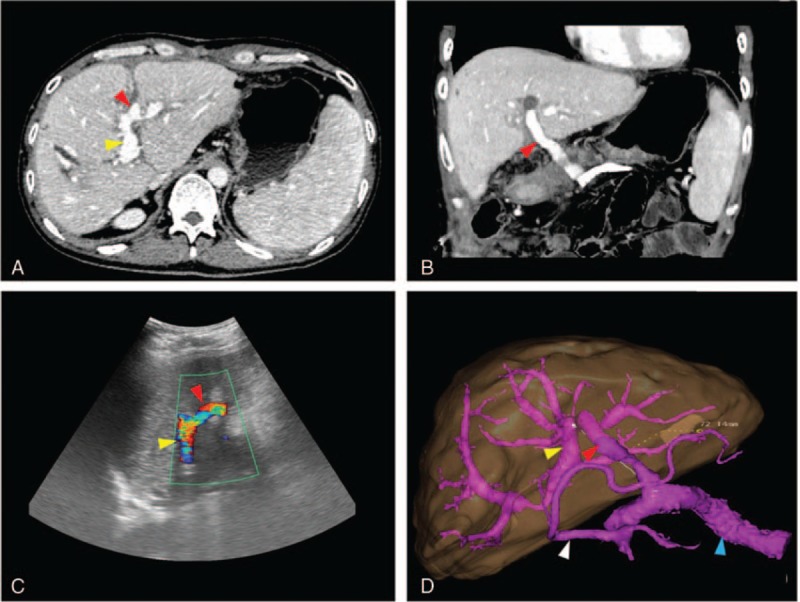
Postoperative enhanced CT scan (A, B) and Doppler US images (C) showed a patent LPV (yellow arrow), MRB (red arrow) and SV (blue arrow); the LPV became dilated and the varix improved (white arrow). On 3D reconstruction, the length of the bypass was shown to be 72.14 mm (D). CT  =  computed tomography, LPV  =  left portal vein, MRB  =  Meso-Rex bypass, SMV  =  superior mesenteric vein, SV  =  splenic vein, 3D  =  three dimensional, US  =  ultrasound.
